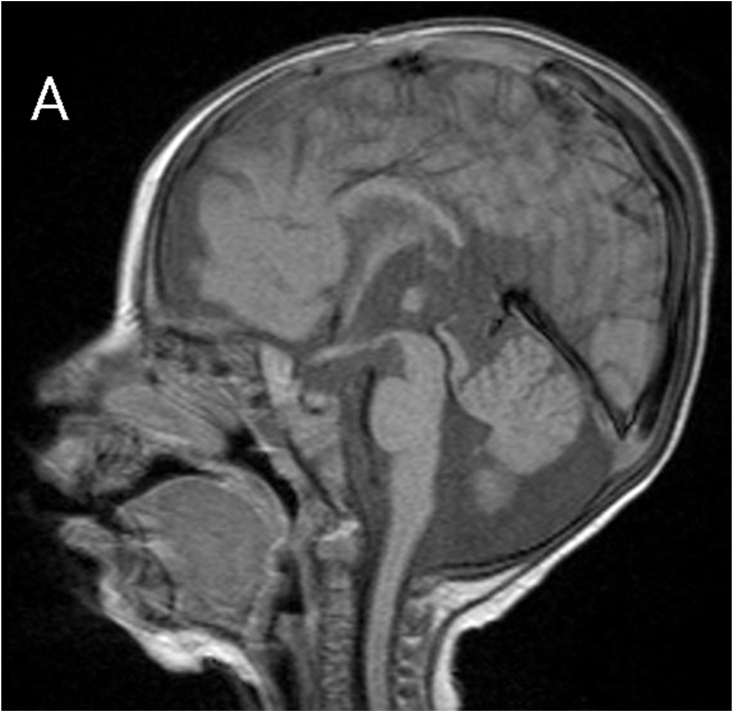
Magnetic resonance imaging (MRI) for our patient at the age of 7 months. A: sagittal T1-weighted image showing hypogenesis of corpus callosum.
